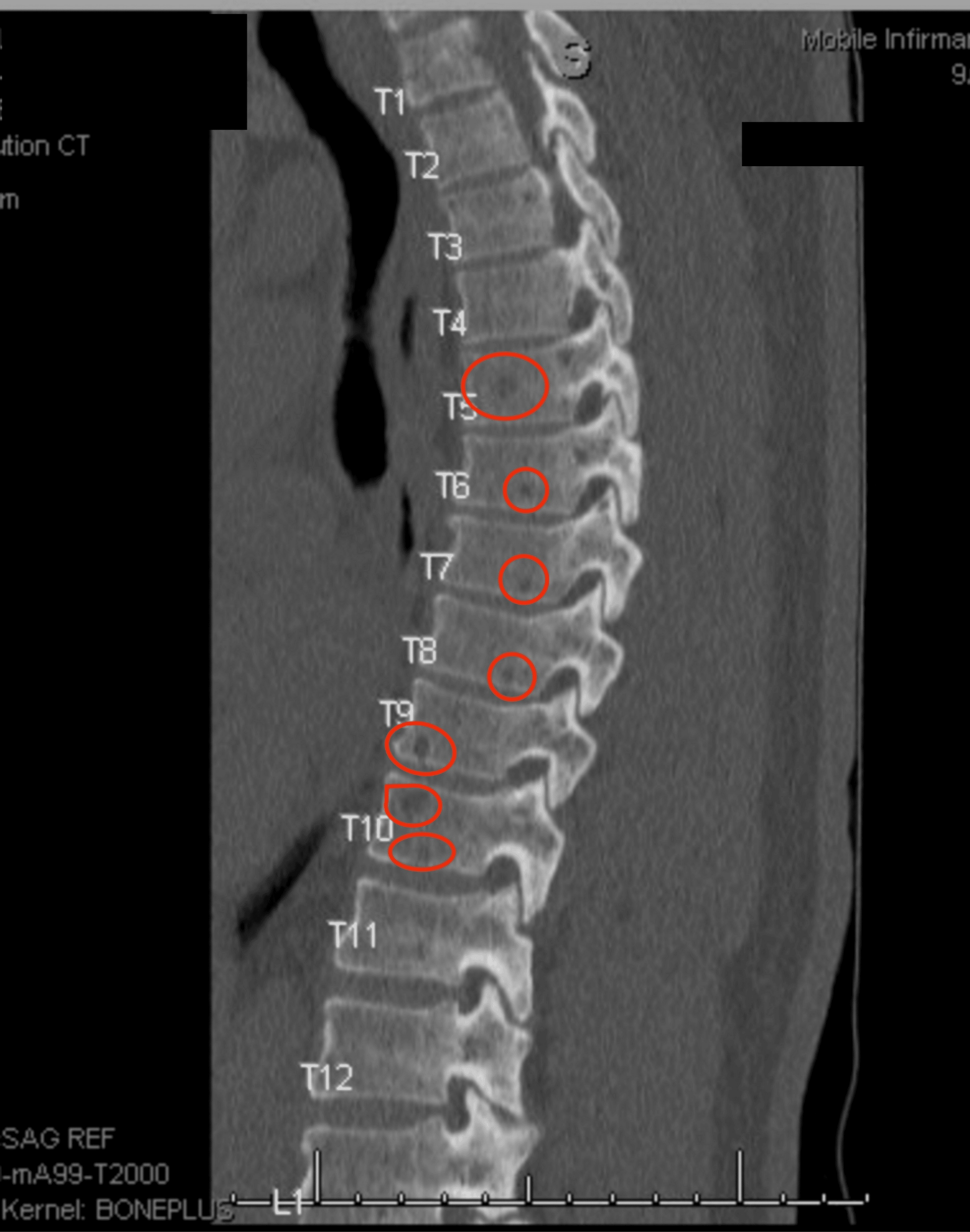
Sagittal CT of the thoracic spine showing multiple lytic lesions, without compression fractures, subluxation, or any paraspinal masses
